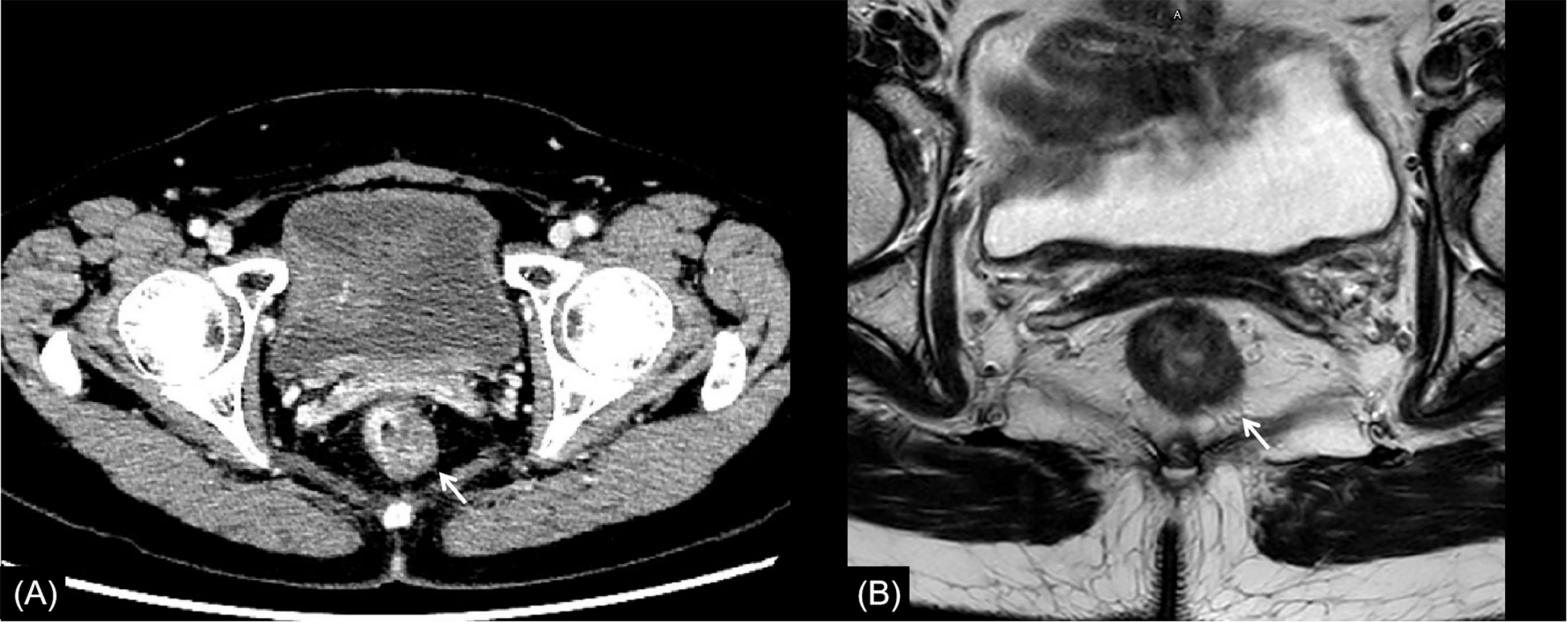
Rectal cancer with ypT2N0. (A) Axial MSCT depicts a heterogeneous enhancing rectal tumor (arrow) with a mild rough outer wall. Over restaged as T3. (B) MRI transverse high-resolution T2WI shows a heterogeneous signal mass involving left rear mesorectal fascia (arrow). Over restaged as T3. yp: pathological staging after neoadjuvant chemoradiotherapy; T, tumor; N, node; MSCT, multi-slice computed tomography; MRI, magnetic resonance imaging.
